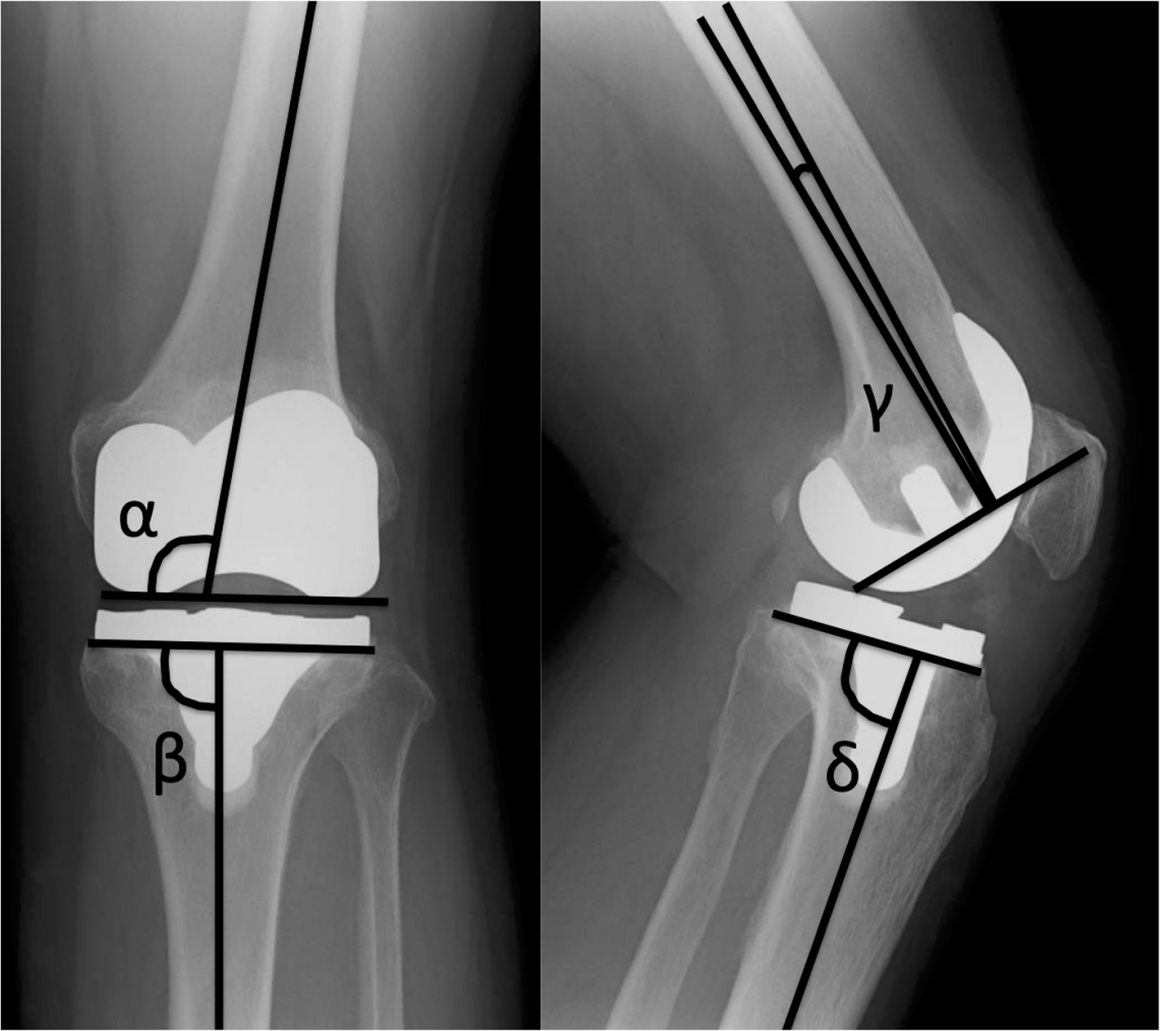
The two-dimensional (2D) post-operative evaluation of component position was performed using standard antero-posterior (AP) and lateral short-knee radiographs. In the AP view, component positions were measured using alpha (α) and beta (β) angles. In the lateral view, component positions were measured using gamma (γ) and delta (δ) angles
